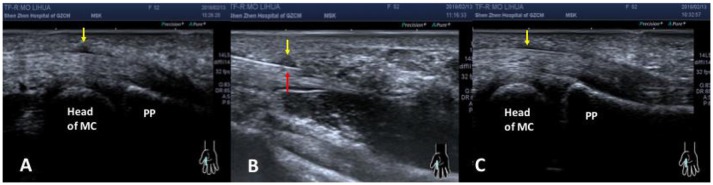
The ultrasound images before (A), during (B) and after (C) release with ultrasonography-guided. (A) The yellow arrow showed the thickening of A1 pulley in the right index finger before operation. (B) The red arrow showed the needle knife was cutting the A1 pulley (yellow arrow). (C) The A1 pulley (the yellow arrow) became normal thickness immediately after ultrasonography-guided release. PP, proximal phalange; MC, metacarpal bone.
